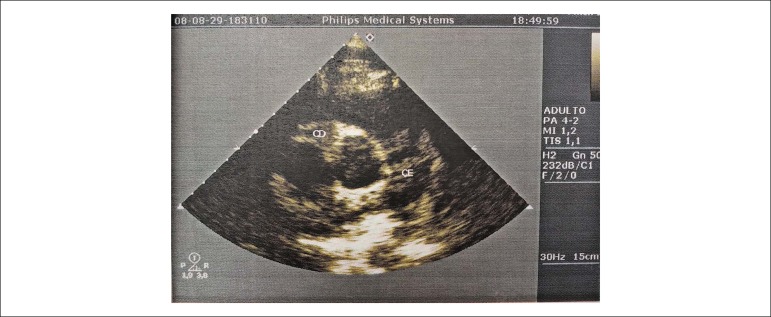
Evident dilation of right coronary artery on transthoracic echocardiogram at rest.
